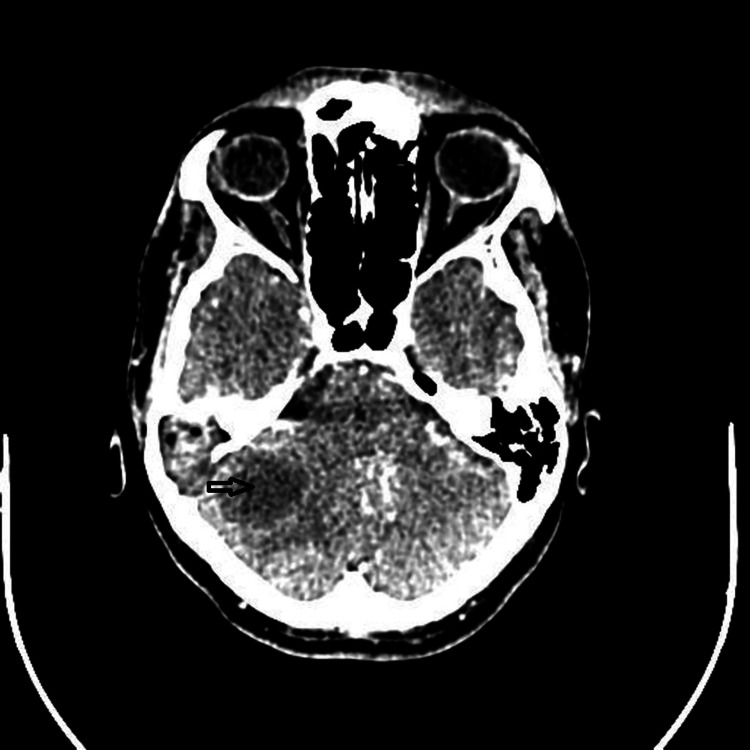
CT brain (axial section) The arrow shows hypodensity in the right hemisphere of the cerebellum
